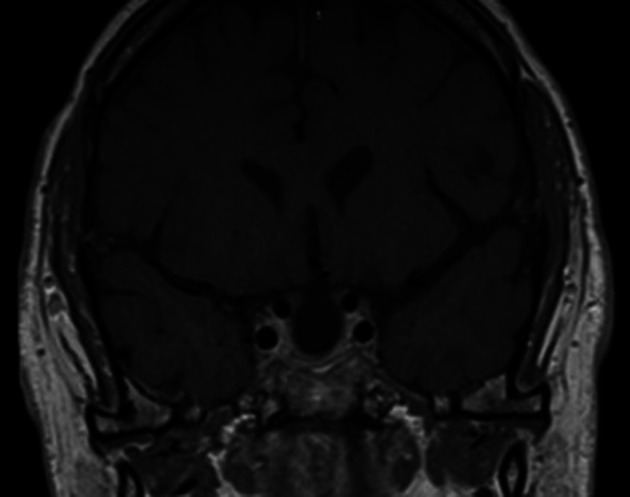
Postoperative follow-up magnetic resonance imaging after 3 months shows near complete resection of the tumour.
